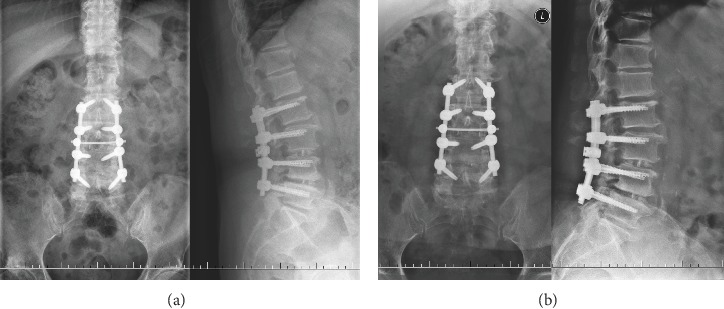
Postoperative anterior-posterior and lateral lumbar radiographs (a). Anterior-posterior and lateral lumbar radiographs of one-month follow-up (b).
